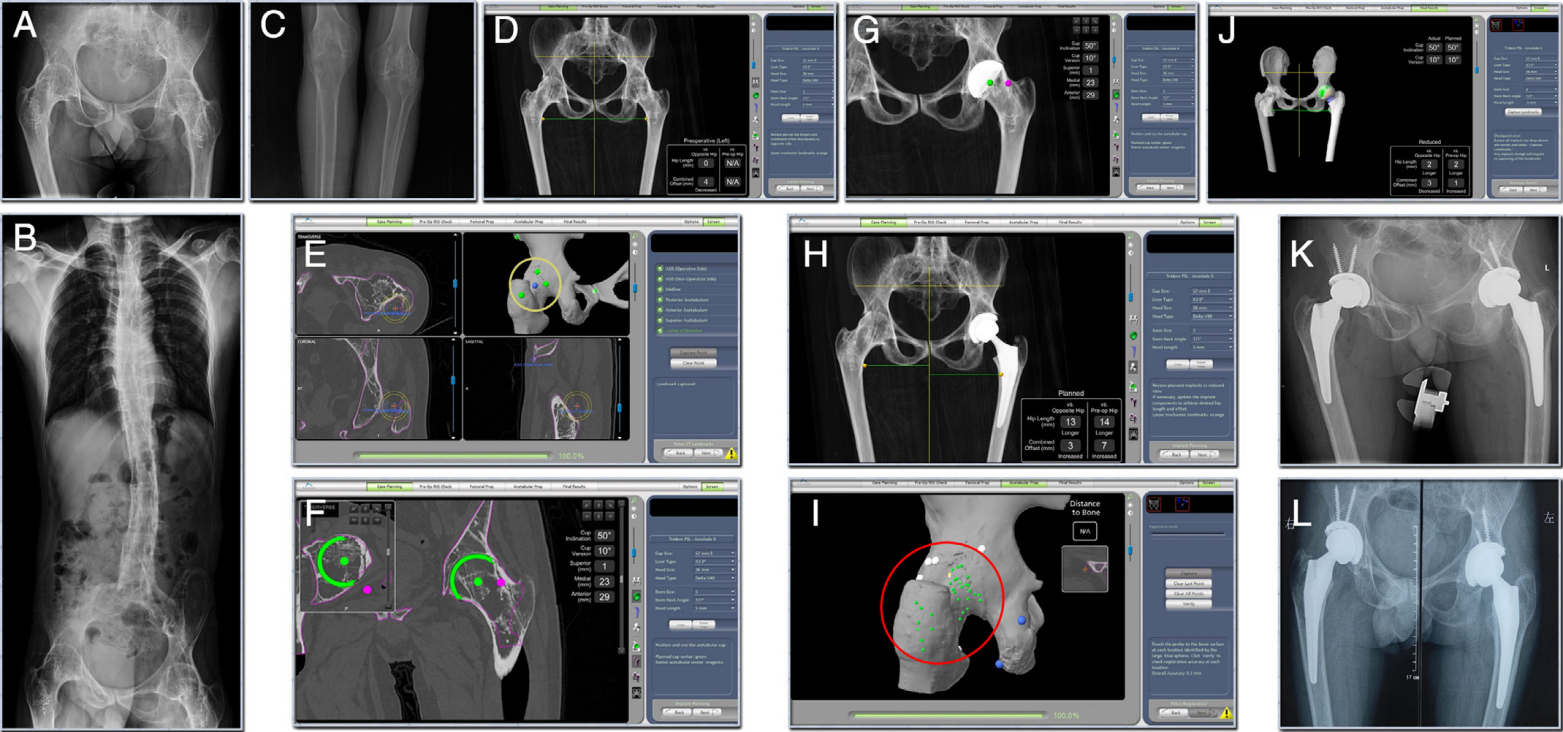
(A) & (B) & (C) Pre‐operative radiographs. Bilateral hips and knees have fused because of ankylosing spondylitis. (D) & (E) & (F) & (G) & (H) CT based pre‐op planning. The 3 acetabular align points that should had been set at the acetabulum were set at the great trochanter and acetabular rim (yellow circle). (I) & (J) & (K) Robotic arm was used to execute that plan. In the registration step of the operation, the circumference of the joint (great trochanter, femoral neck, acetabulum rim) was registered instead of the acetabulum (red circle). The robotic arm assisted THA had achieved the surgery plan. (l) Radiograph 3‐months post‐operative showed no change in the position of the prosthesis.
